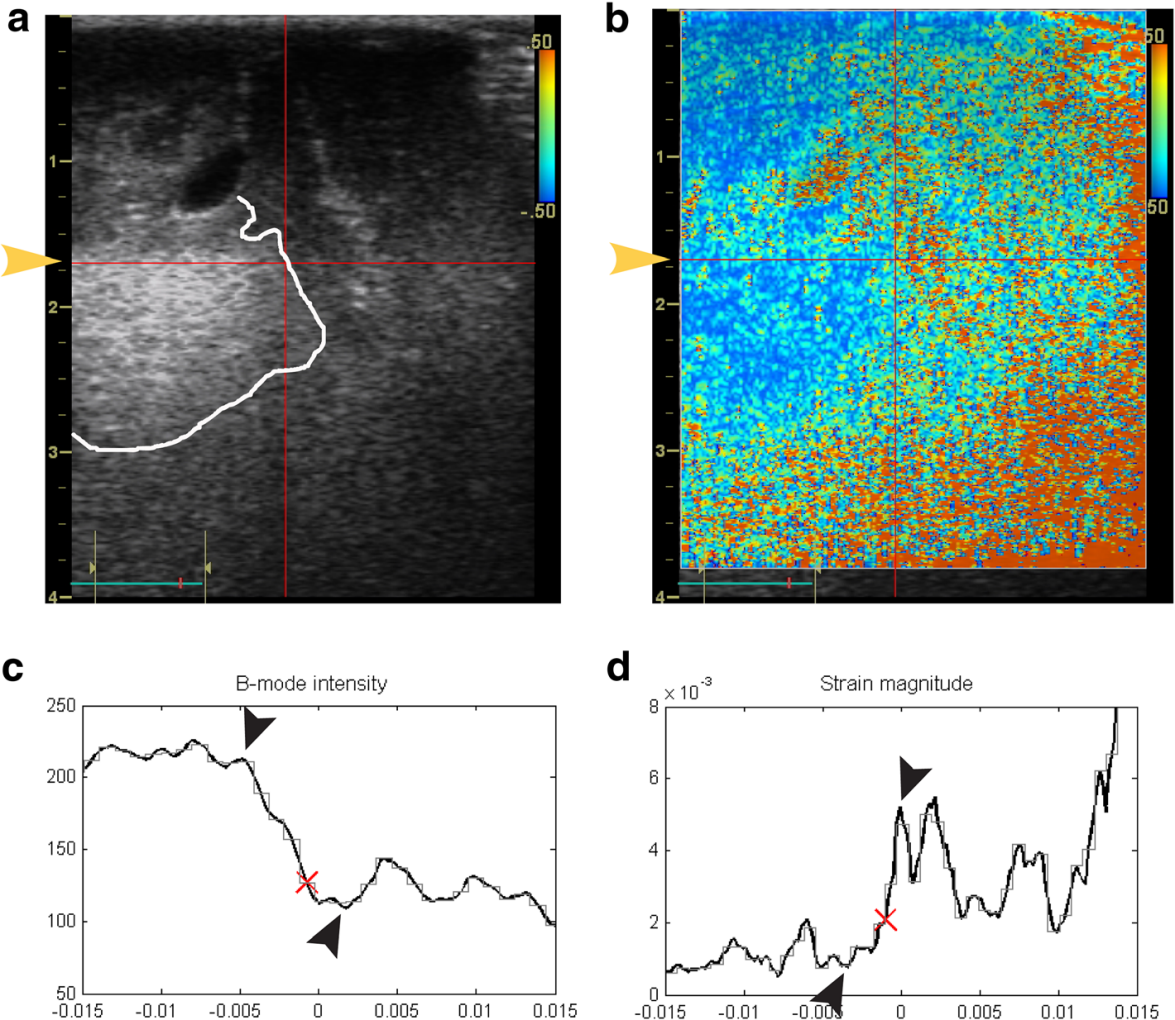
Ultrasound B-mode and strain images. The ultrasound B-mode image of a glioblastoma (grade IV) in (a) and the corresponding strain magnitude image (b). For display purposes the delineation of the solid tumour close to the location of the measurements is marked with a bright line in the B-mode image. The B-mode intensity plotted along the lateral direction at a user- selected depth is shown in (c), with the numbers of the x-axis referring to the distance [m] from the lateral midpoint of the image. The strain magnitudes are plotted in similar way and displayed in (d), with the Y-axis showing strain magnitude values from 0–8 ‰. The depth of the plotted magnitudes is for display purposes indicated with a bright arrow in (a) and (b). The values picked for estimation of contrast between tumour tissue and assumed normal tissue are indicated by black arrowheads in (c) and (d).
